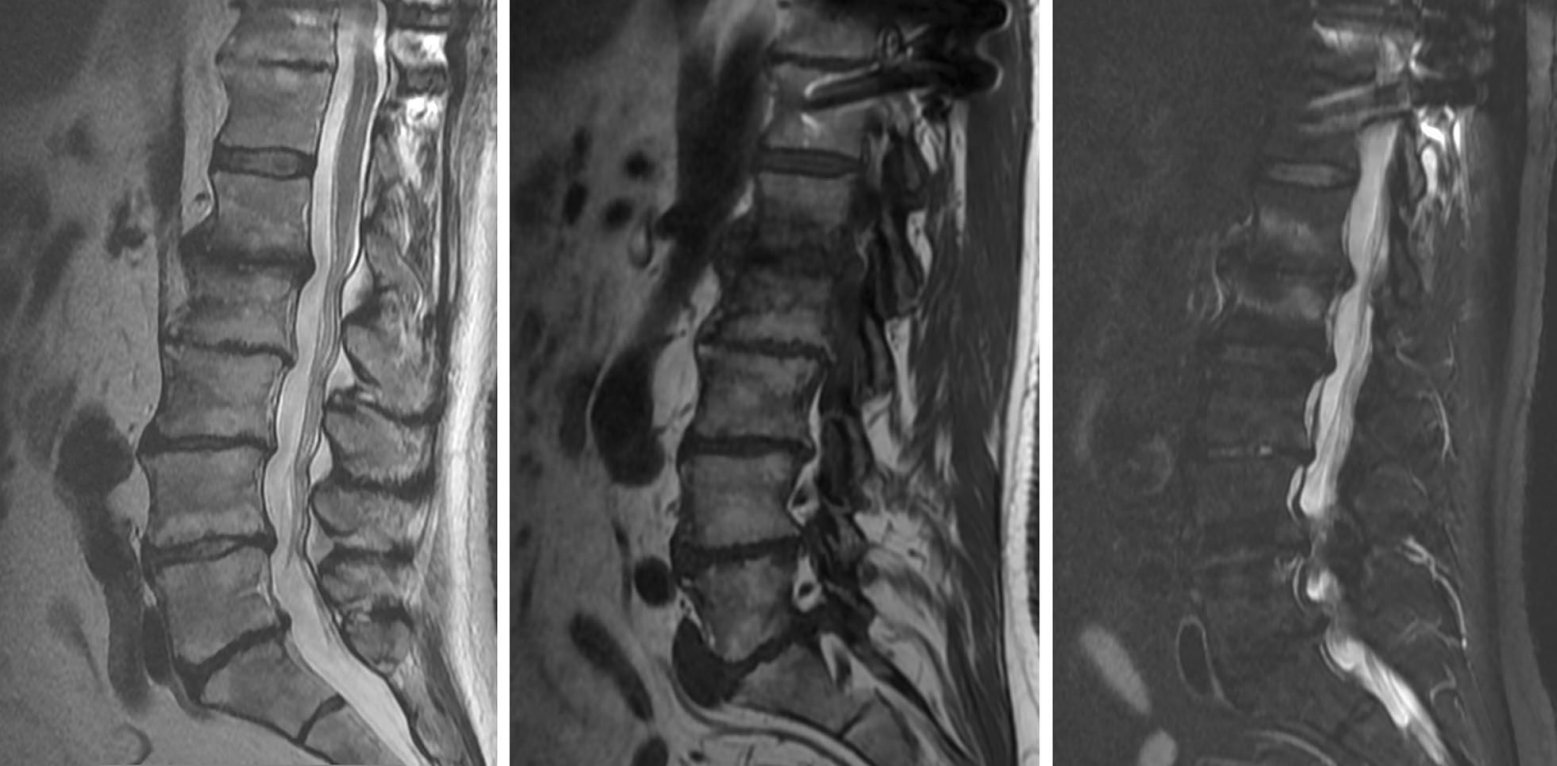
Magnetic resonance imaging (MRI) of the lumbar spine. *Left* sagittal T2-weighted, *center* sagittal T1-weighted, and *right* sagittal T2 with fat suppression images, confirming the advanced degenerative changes, but without any critical central canal stenosis (technique: sagittal images were obtained with fast-spin echo T1-weighting, T2 weighting, and T2 with fat suppression. Axial fast-spin echo T2 weighted images were also obtained)
